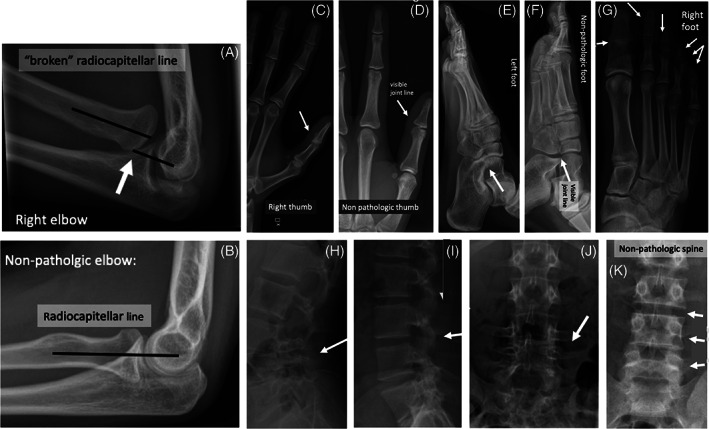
Radiographs showing the present patient with SYNS3 and a missense variant in FGF9. (A) Radial head dislocation of the elbow; (B) Normal elbow; (C) Synostosis of the interphalangeal joints of the thumb; (D) Normal thumb; (E) Suspected calcaneonavicular coalition of the feet; (F) Normal foot; (G) Synostosis of dig I and of the distal interphalangeal joints of dig II‐V of the feet; (H) Synostosis of the dorsal structures of the lumbar spine; (I, K) Well defined vertebrae and intervertebral joints of the lumbar spine and (J) Reduced height of the disc L3‐L4 and L4‐L5
